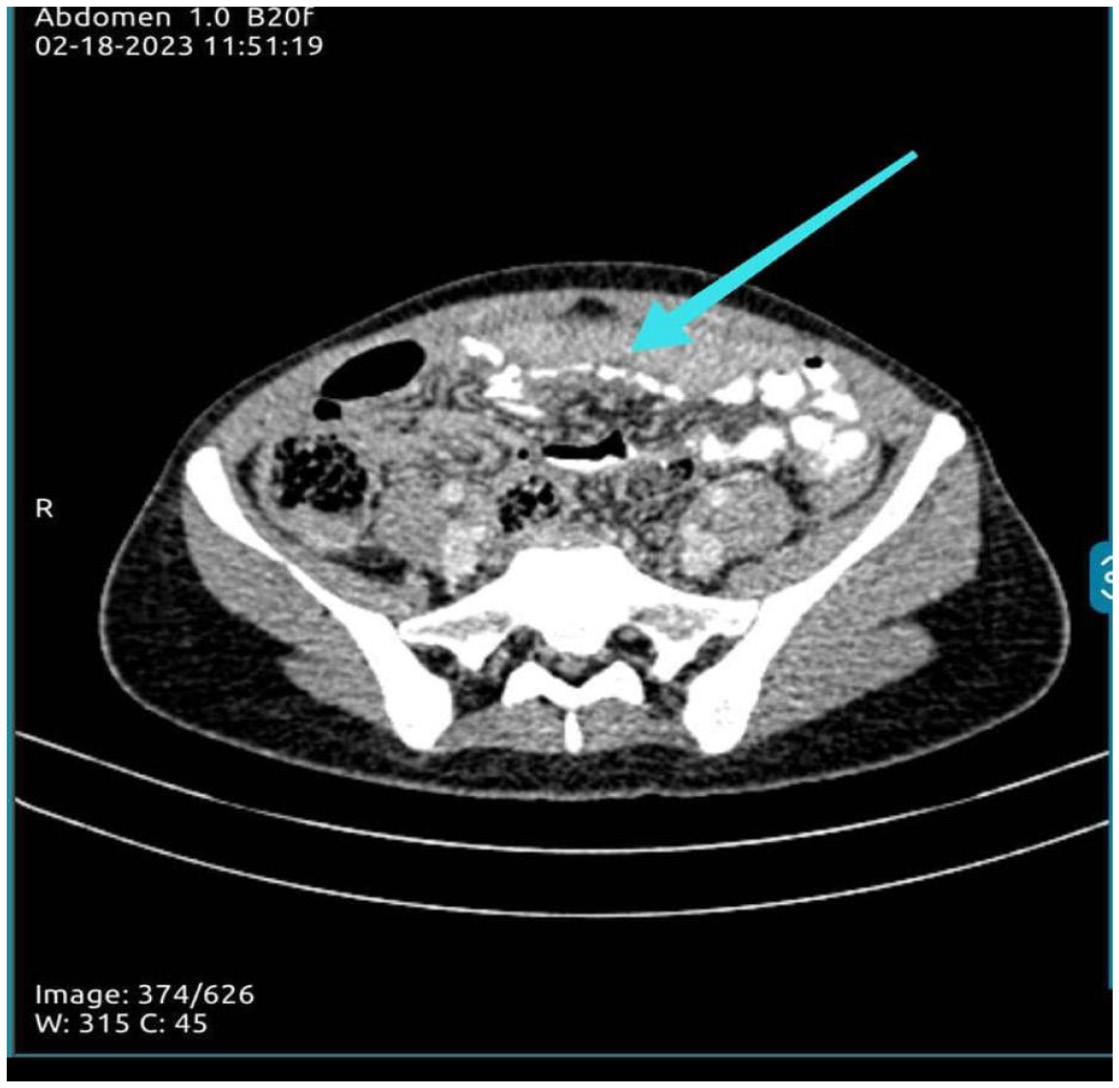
Cross‐sectional abdominal CT scan image showing evidence of diffuse peritoneal thickening with diffuse thickening of the small bowels with multiple nonspecific enlarged lymph nodes.
